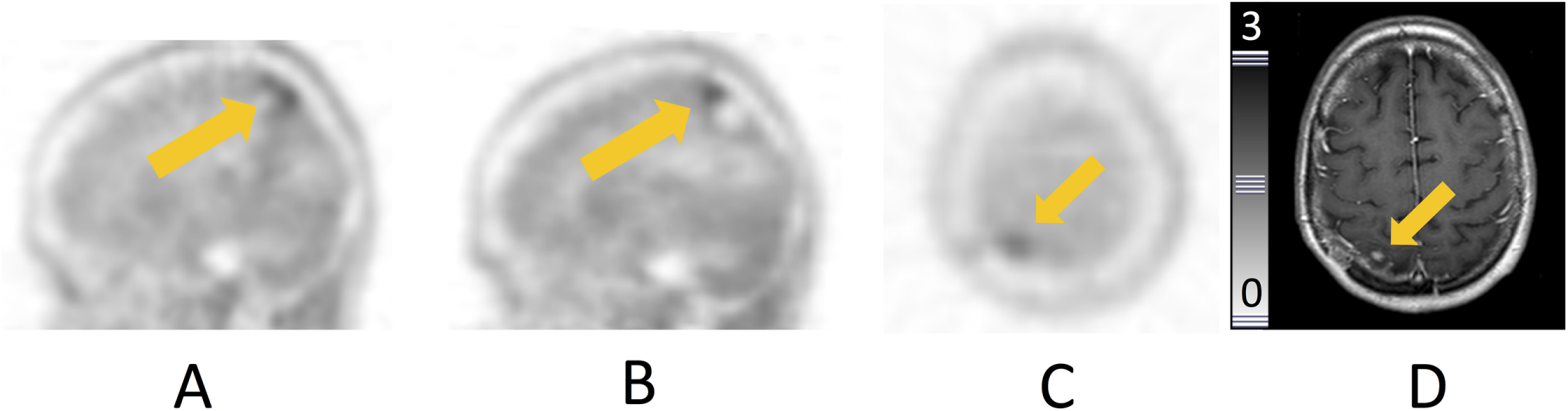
Residual tumor tissue detected by [^18^F]FET-PET—Follow-up. Patient #18. A,B: sagittal slices. C,D: axial slices. A: Pre-operative PET showing focal uptake in the right parietal lobe. B: Suspicious uptake in the cranial border of the resection cavity 48h and C: 4 months after resection, consistent with vital tumor. D: Corresponding MRI 4 months after resection corroborating the PET finding.
